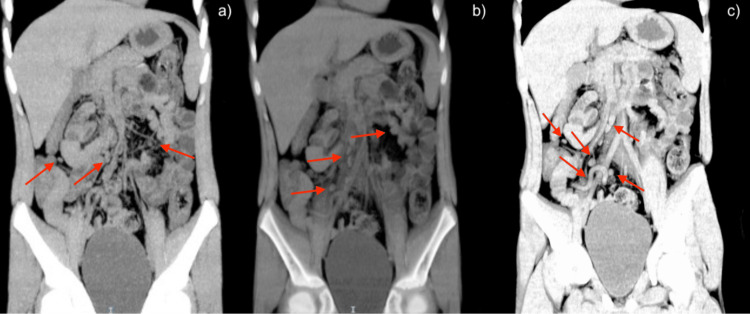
Coronally sectioned abdominal CT scan. (a, b) Findings consistent with mesenteric adenitis. Multiple oval-shaped images correspond to swollen mesenteric lymph nodes. More than five mesenteric lymph nodes are observed with short axes exceeding 10 mm. (c) Retrocecal appendix of increased density, with a maximum transverse diameter up to 4.4 mm at the base, without peripheral inflammatory changes, and with the presence of a small appendicolith at the tip measuring more than 2 mm in diameter. CT: computed tomography
